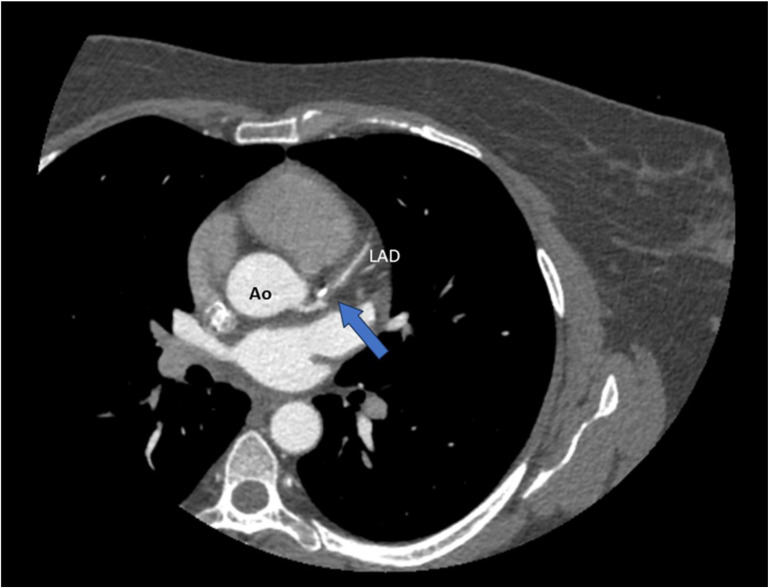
CT coronary angiography (CTCA) revealing a spontaneous dissection of the left anterior descending artery (LAD) (blue arrow). Ao = aorta.
